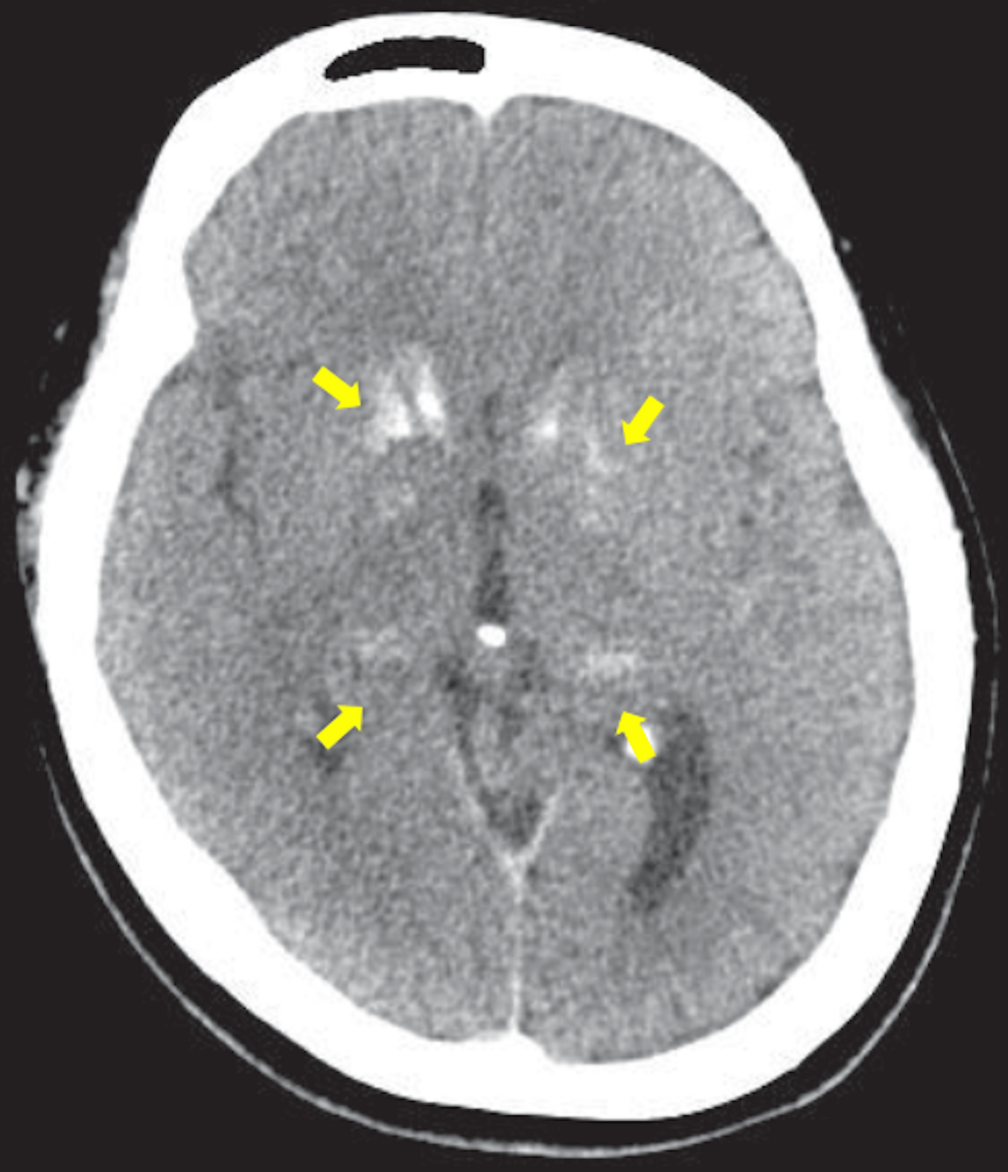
Non-contrast head CT at age 21 A non-contrast head CT reveals new calcifications (yellow arrows) located in the basal ganglia and thalamus, corresponding to the hyperintense regions observed on MRI. The interval appearance of calcifications underscores the progressive nature of structural changes, which were not present in childhood imaging.
